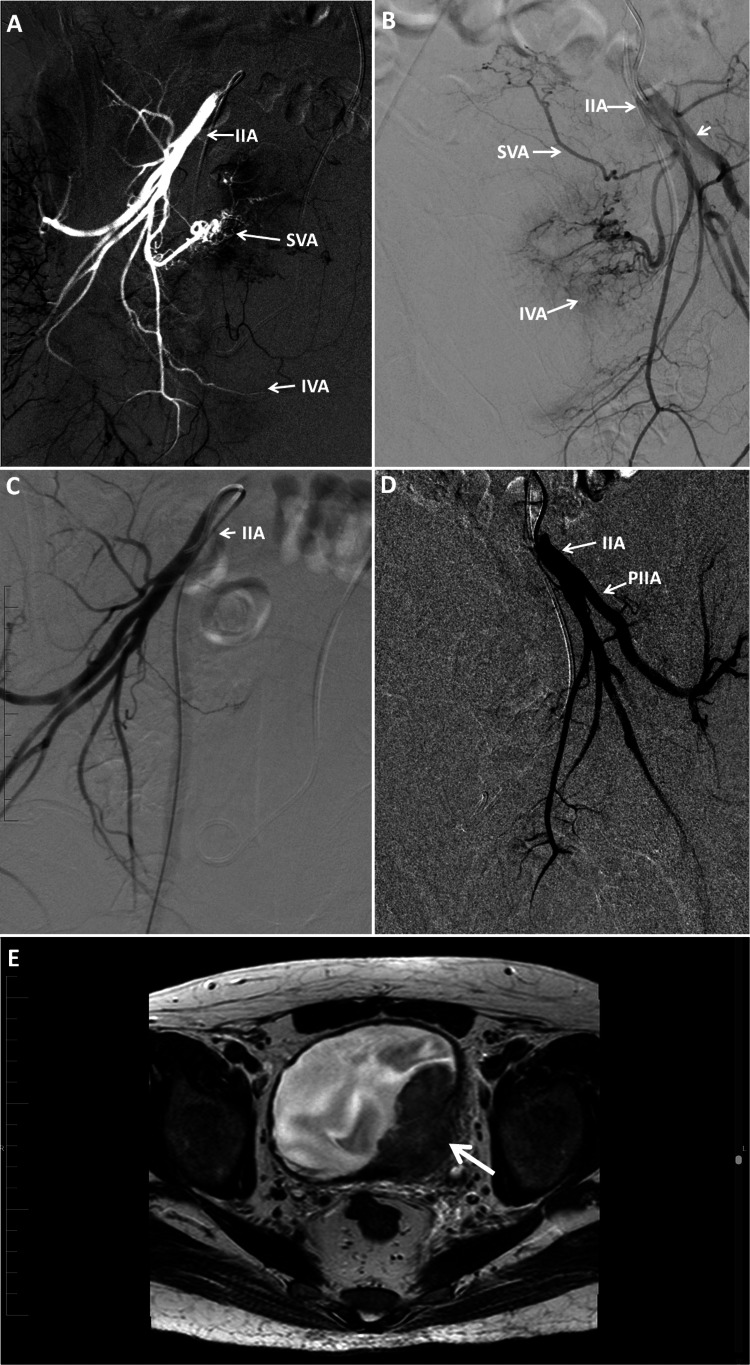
Patient 1: (A) right angiogram showing hypervascularity. (B) Left angiogram showing hypervascularity. (C) Right angiogram post-embolization showing a reduction in blood flow to the vesical arteries. (D) Left angiogram post-embolization showing cessation of flow to the vesical arteries. (E) MRI with a large tumor involving the posterolateral bladder wall with peri-vesical fat invasion. IIA, internal iliac artery; PIIA, posterior branch of internal iliac artery; SVA, superior vesical artery; IVA, inferior vesical artery; MRI, magnetic resonance imaging
